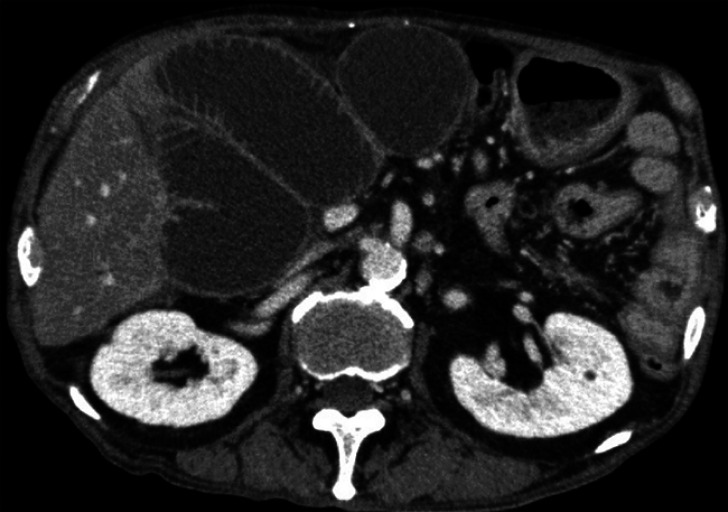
Computed tomography showed a dilated afferent loop due to a malignant stricture.
